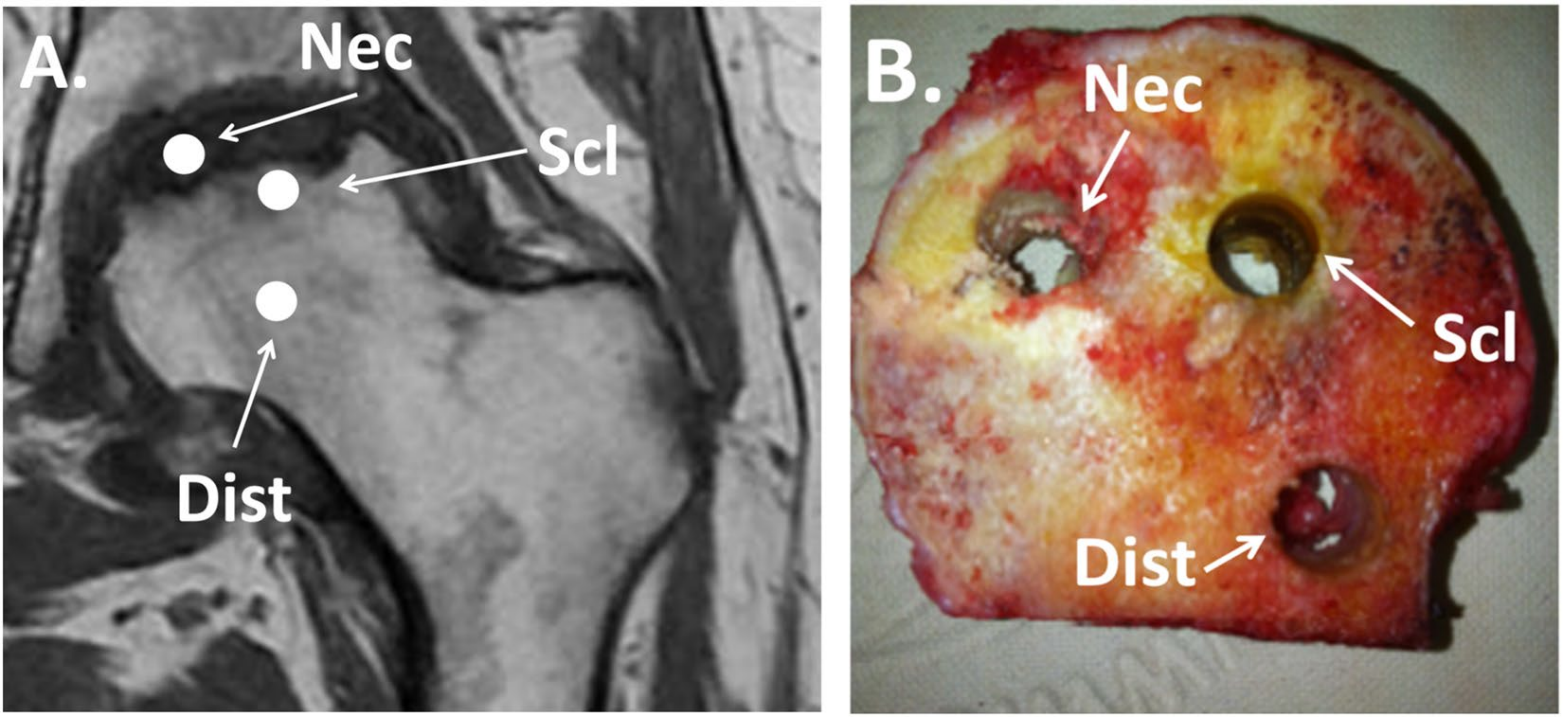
Magnetic resonance imaging (A) and macroscopic (B) localizations of the three sampled zones. Nec = necrotic zone, Scl = sclerotic zone, Dist = distant zone.
